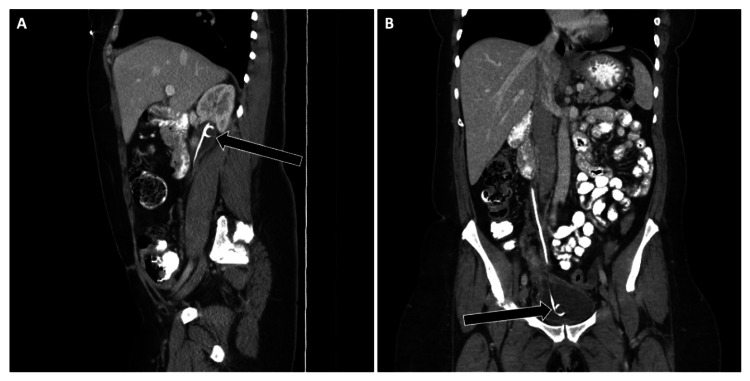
Correct Positioning of a Double-J Ureteral Stent Contrast-enhanced CT of the abdomen and pelvis demonstrating appropriate placement of a right double-J ureteral stent.
